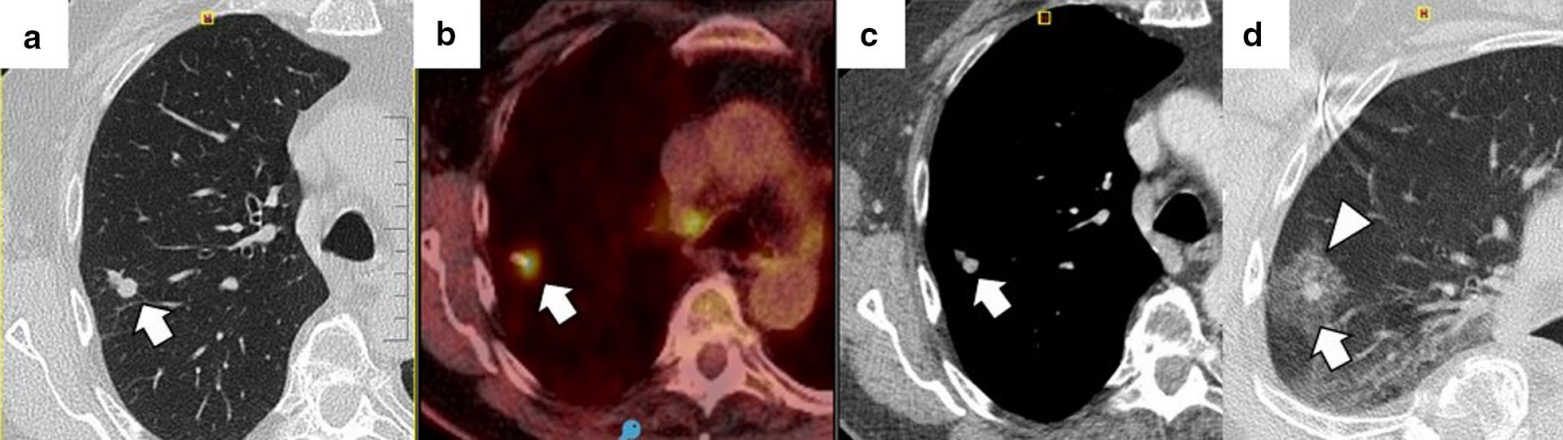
Diagnosis and retreatment of a tumor recurrence previously treated by image-guided percutaneous pulmonary RFA. a–c Appearance of a solid nodular lesion adjacent to an ablative area in the radiologic control performed six months after a percutaneous lung RFA to treat lung metastasis from a rectal carcinoma (arrow). This lesion exhibits avid FDG (b) and iodine contrast (c) uptake (arrows), and it is consistent with a tumor recurrence at the ablation site. d The lesion is re-treated using percutaneous lung MWA, with a central consolidative area (arrow) surrounded by a ground-glass halo of more than 5 mm (triangle). These findings are consistent with comprehensive treatment
